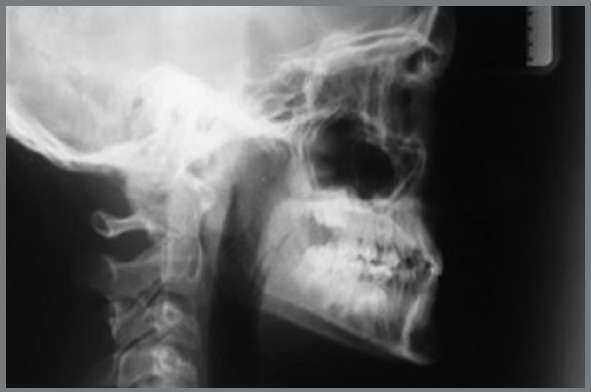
Lateral radiograph at 2-year follow-up.
